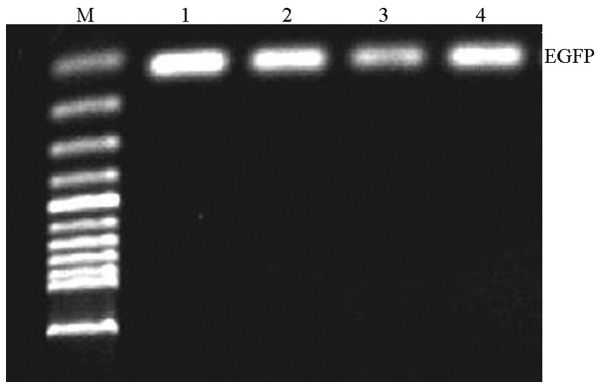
EGFP cDNA electrophoresis results for the synovial tissues of all four experimental groups. M, marker; 1, EGFP plasmid only group; 2, ultrasound + EGFP plasmid group; 3, microbubble + EGFP plasmid group; 4, ultrasound + microbubble EGFP plasmid group; EGFP, enhanced green fluorescent protein.
